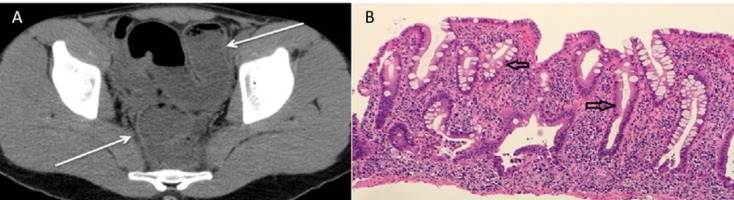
CT Scan (Figure A) and Intestinal Biopsy (Figure B) Figure A: Computed tomography (CT) scan demostrating mild wall thickness and featurelesss appearance of rectosigmoid colon Figure B: Descending colon at four times visulaization showing crypt distortion, crptitis and crypt abscess.
